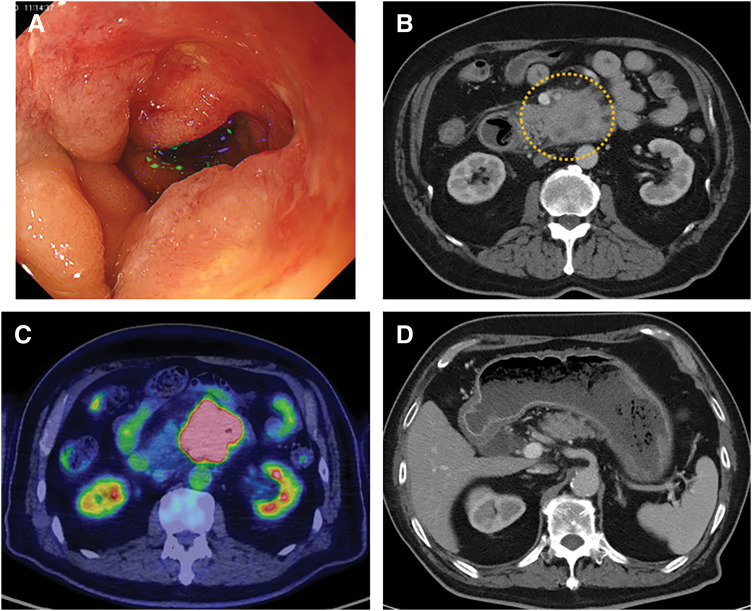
Preoperative imaging and endoscopic findings. (A) Upper gastrointestinal endoscopy revealed a circumferential, irregular ulcerative lesion in the 3rd portion of the duodenum, causing luminal narrowing. (B) Contrast-enhanced CT demonstrated an approximately 50-mm, irregularly marginated mass in the 3rd portion of the duodenum. (C) PET-CT showed increased fluorodeoxyglucose uptake in the 3rd portion of the duodenum, consistent with the tumor site. (D) Axial CT showed dilation of the stomach and proximal duodenum due to obstruction.
